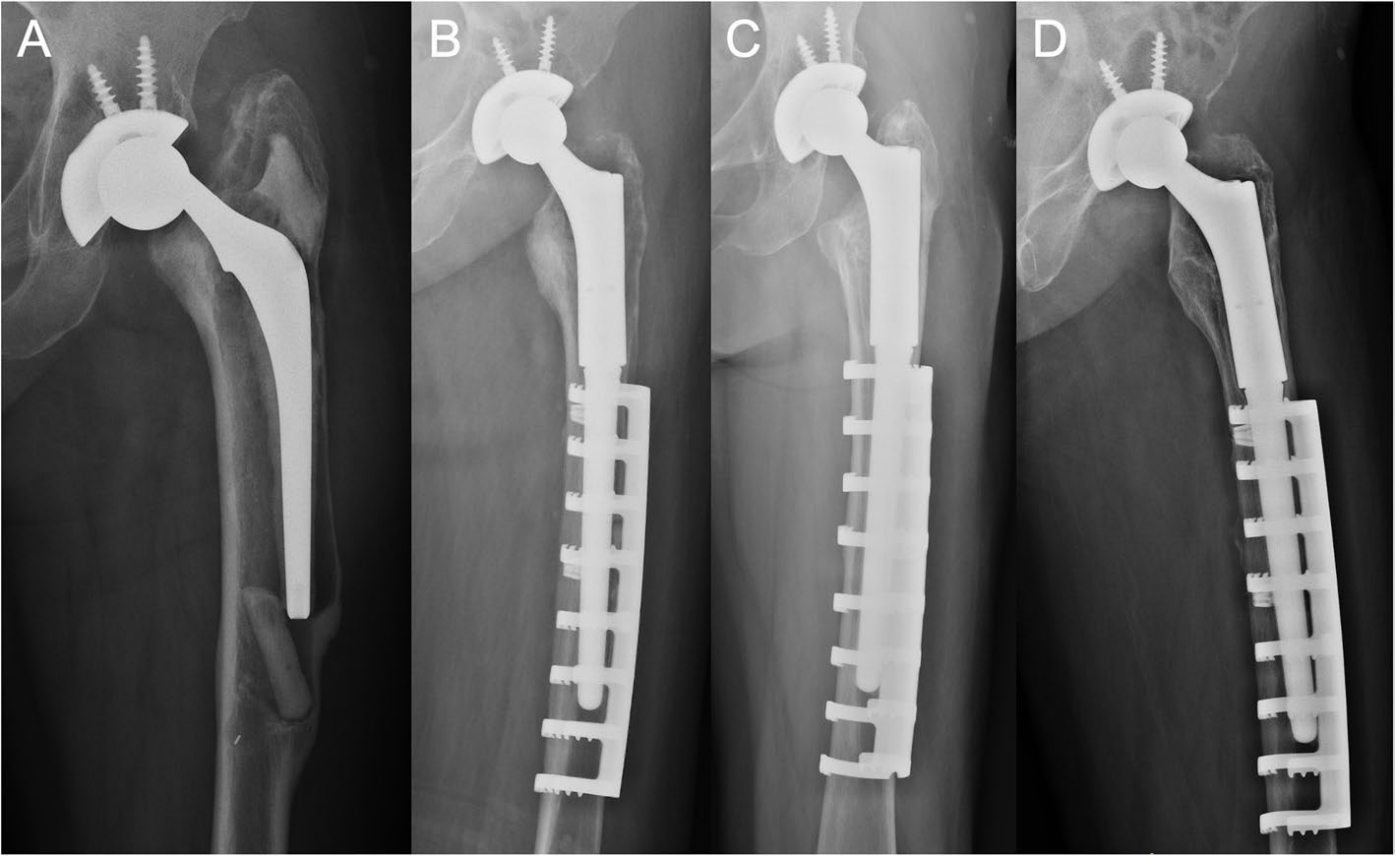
A 71-year-old female patient experienced left aseptic loosening 10 years after primary hip arthroplasty. A Preoperative radiograph. B Immediately after surgery. C 3 years after surgery. No evident stress shielding was detected. D 8 years after surgery, there was obvious cortical and diaphyseal bone resorption in the proximal medial femur
